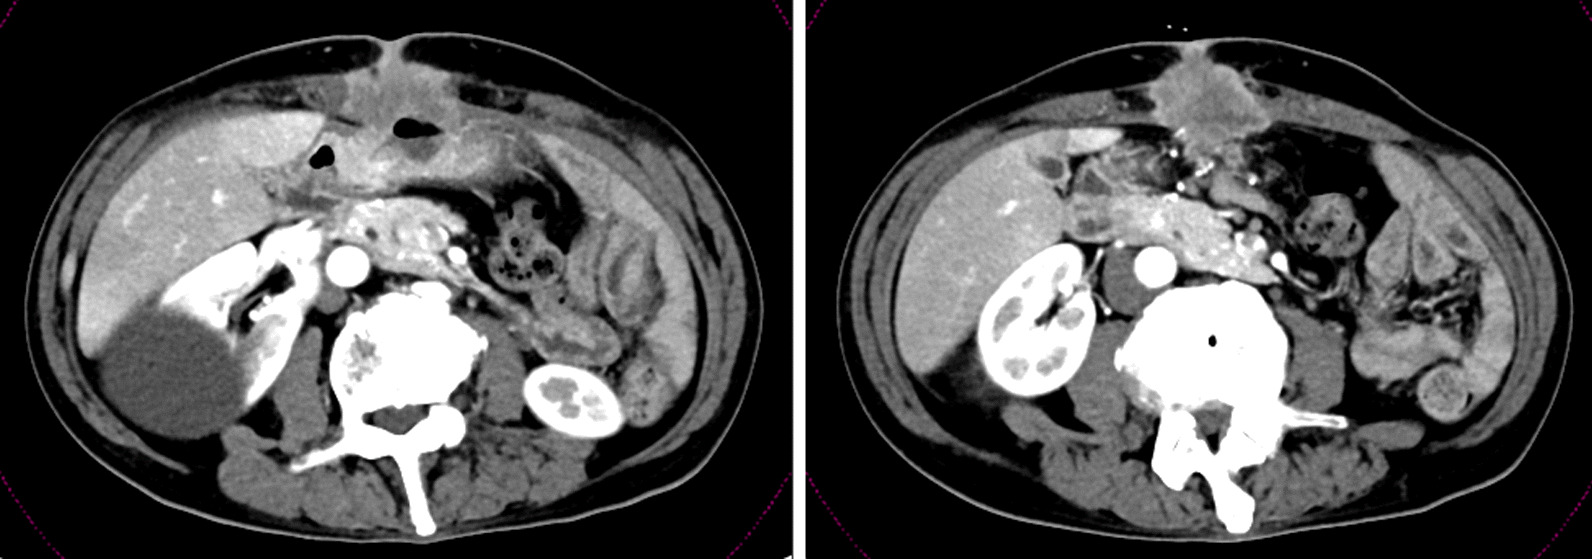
Pretreatment contrast-enhanced computed tomography scan showing the primary gastric tumor with massive invasion of the abdominal wall and enlarged peritumoral lymph nodes
